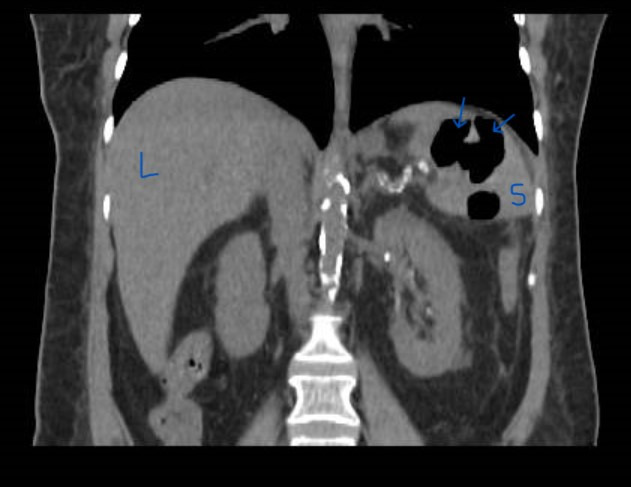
CT with intravenous contrast revealedlarge area of hypoattenuation within the spleen, mainly in the peripheral region that was suggestive of splenic infarction with signs of splenic abscess. L liver, S spleen.
